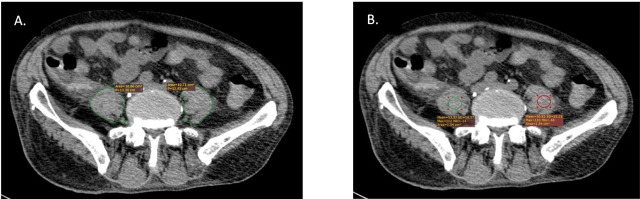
CT psoas muscle evaluation at the third lumbar vertebrae. A. Cross-sectional area evaluation showing muscle area. B. Evaluation of muscle density in Hounsfiled unit.
